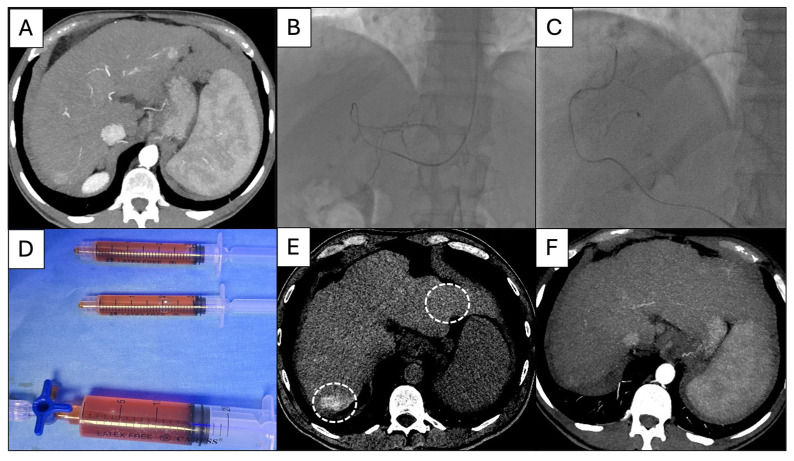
DSM-TACE with idarubicin performed according to a standard protocol. A 75-year-old male affected by HCV-related cirrhosis and HCC BCLC B. (A) In CT, 3 hypervascularized HCC nodules in arterial phase were evident in S7, S2 and S5. (B,C) Via right radial access, a 5 Fr diagnostic catheter (headhunter or multipurpose tip) was advanced in the common hepatic artery and then a 2.4 Fr microcatheter was coaxially positioned superselectively to the nodule feeders. (D) Here 4 mL of DSM was mixed with idarubicin (10 mg Zavedos^®^ diluted in 7 mL of saline) and 9 mL of pure iodinated contrast agent, then injected via 3 mL Luer lock syringes; after chemotherapy, temporary embolization was obtained with 3.5 mL of DSM mixed with 6.5 mL of pure iodinated contrast agent. (E) Plane CT scan acquired in the angio suite immediately after TACE, depicting nodule opacification (white dotted circles). (F) The 3-month follow-up CT in arterial phase revealing net enhancement reduction in the nodule in S7 and disappearance of the nodule in S2, compatible with PR at mRECIST criteria evaluation.
